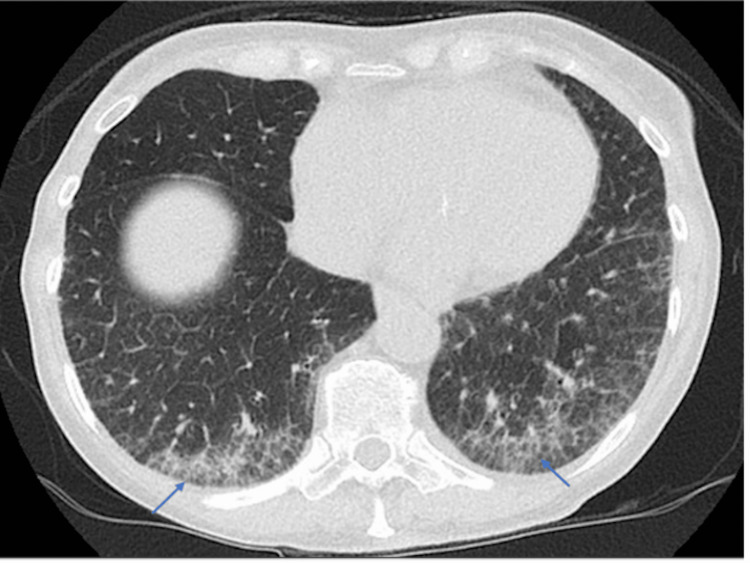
Axial high-resolution computed tomography (HRCT) image of the chest demonstrating interlobular septal thickening and mild subpleural ground-glass opacities in the bilateral lower lobes
